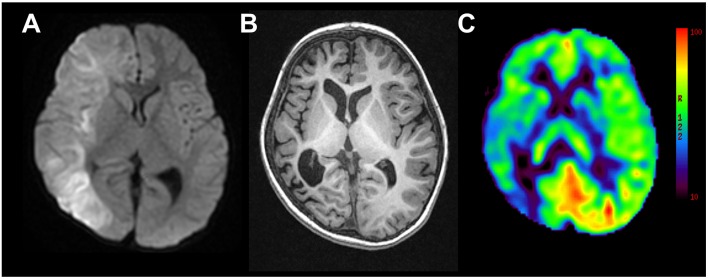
Representative magnetic resonance images from case 2. (A) Diffusion weighted imaging at day 5 following onset of left-sided convulsion revealed high signals in subcortical areas in the right hemisphere. (B) T1-weighted imaging 2 years following onset revealed right hemispheric atrophy. (C) Arterial spin labeling at the same time revealed hypoperfusion in the right hemisphere.
